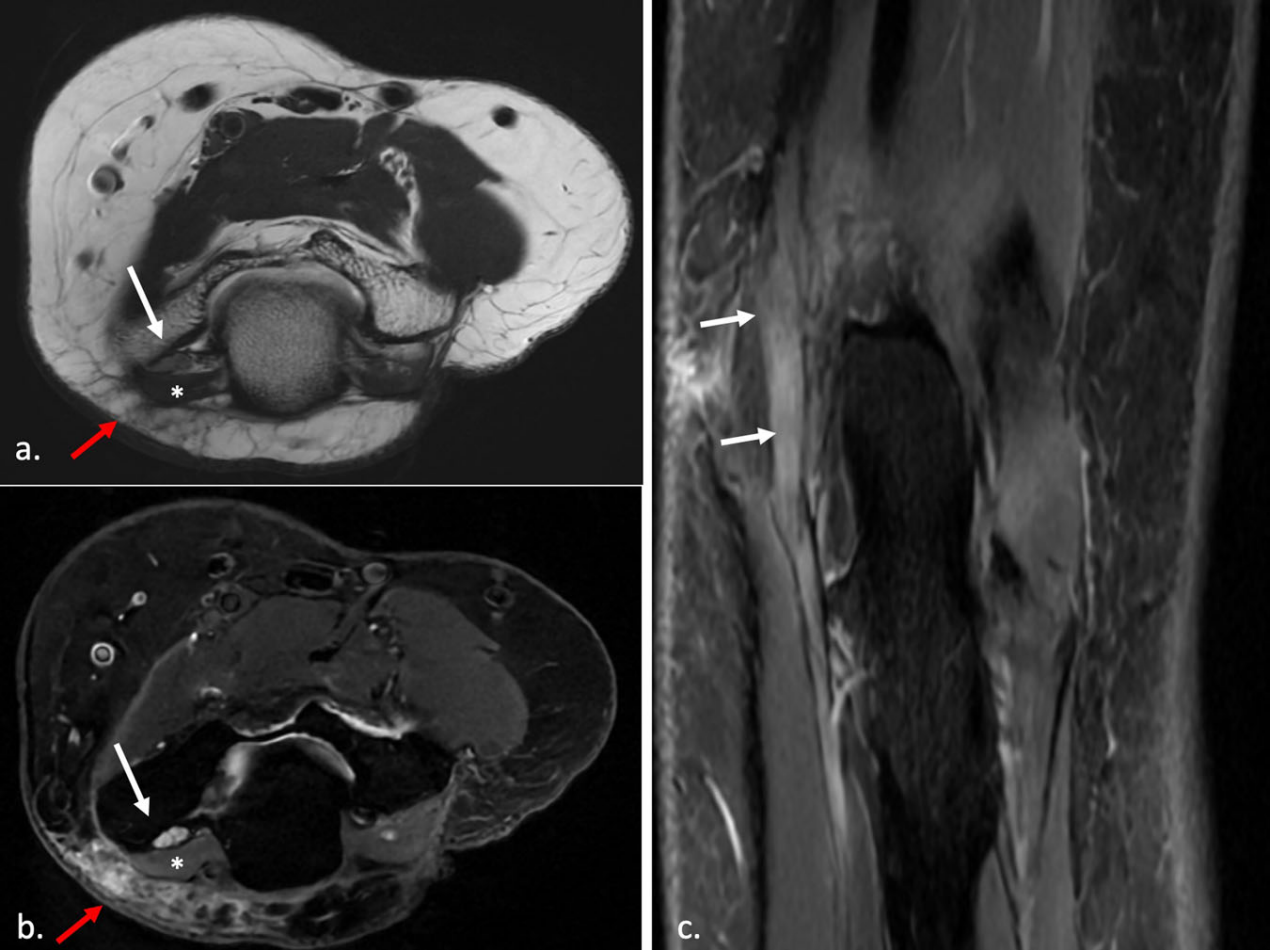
MRI of anconeus epitrochlearis muscle. Axial T1 (a), axial (b), and coronal (c) fat-suppressed PD images show an accessory anconeus epitrochlearis muscle (*), enlargement and hyperintensity of the UN in the cubital canal (white arrow) and edema of the surrounding soft tissues on the posteromedial aspect of the epitrochlea (red arrow)
